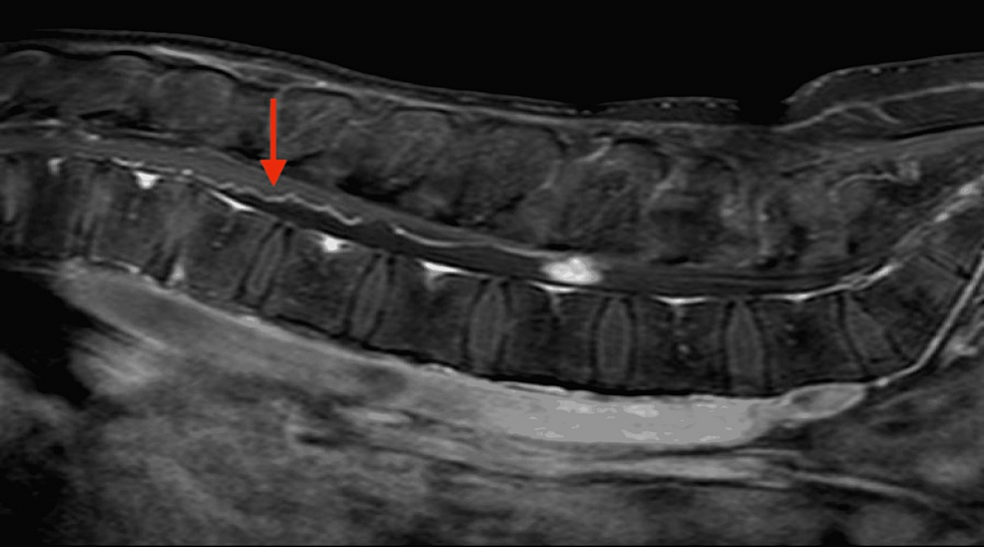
Sagittal T1 Gd+ MRI of the same patient Sagittal T1 Gd+ MRI of the same patient disclosed abnormal, serpiginous vessels between the tumour at L3 and the conus medullaris (arrow). This represents a typical sign of filum terminale paragangliomas.
